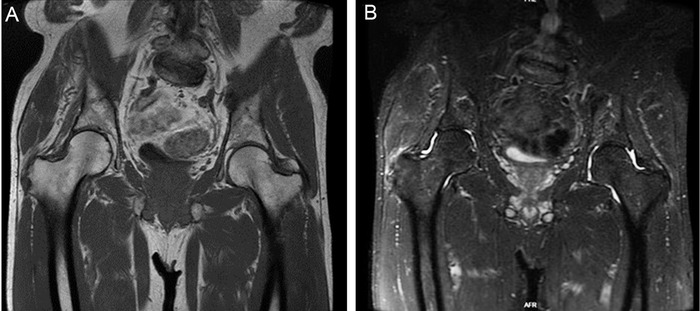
T1 (A) and T2 (B) coronal magnetic resonance images show high-grade retracted tear of the right gluteus medius and gluteus minimus tendon.
